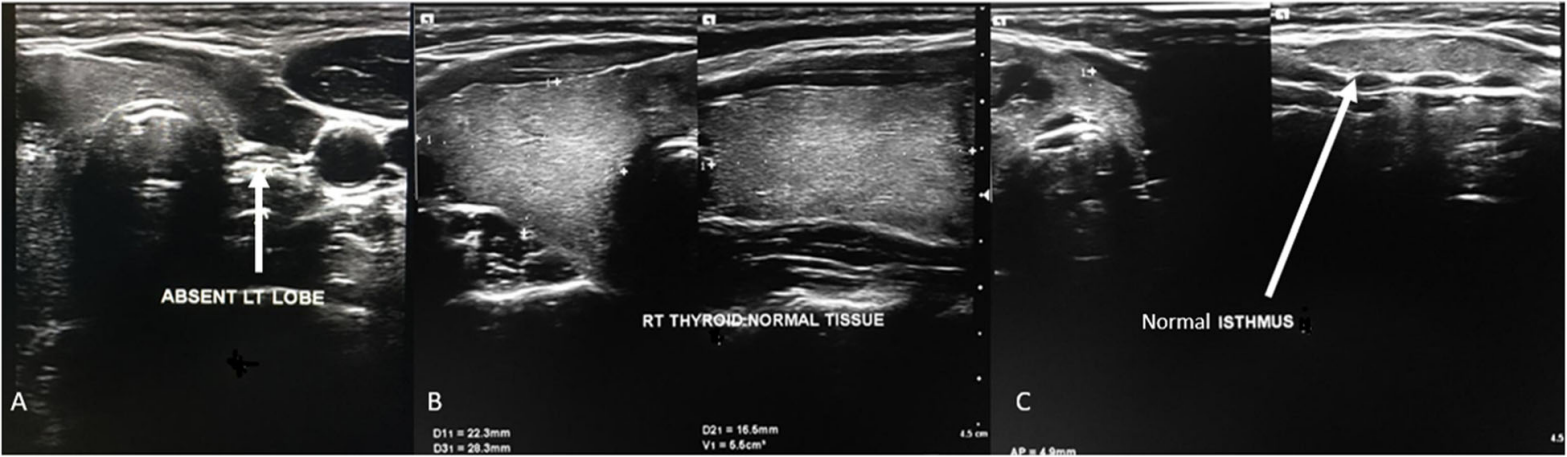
Ultrasound of the thyroid gland with high frequency linear probe  demonstrating a Absent left thyroid lobe b The right thyroid lobe normal tissue volume, and  c Isthmus in situ both in longitudinal and transverse planes
